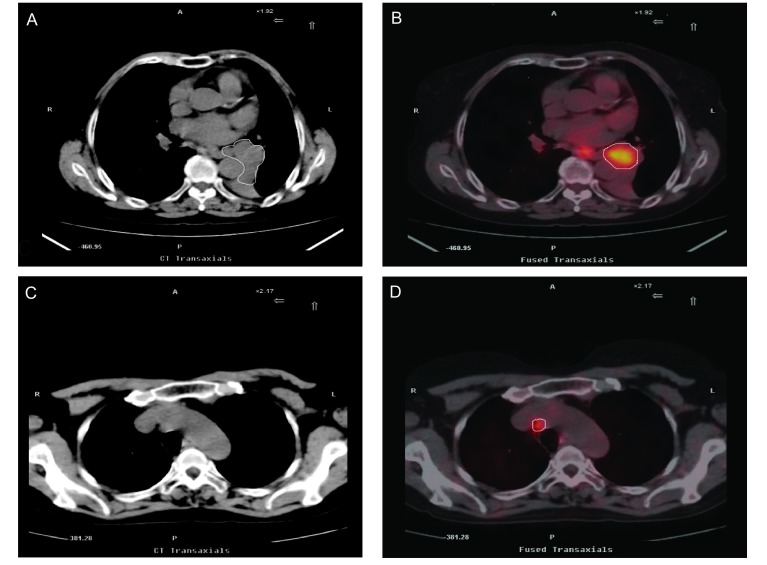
^18^F-FDG-PET/CT显像对NSCLC的靶区体积的影响。A：CT示左肺下叶癌并肺不张；B：融合图像区分了不张与肿瘤组织；C：CT示无明确的转移淋巴结；D：PET-CT发现相应部位淋巴结放射性浓聚。 The Influence of PET-CT image on tumor volume of NSCLC. A: CT showed primary tumor complicated with atelectasis of left lower lobe of the lung; B: PET-CT showed activity uptake of gross tumor; C: CT showed negative mediastinal lymph node; D: PET-CT showed activity uptake of mediastinal lymph node.
